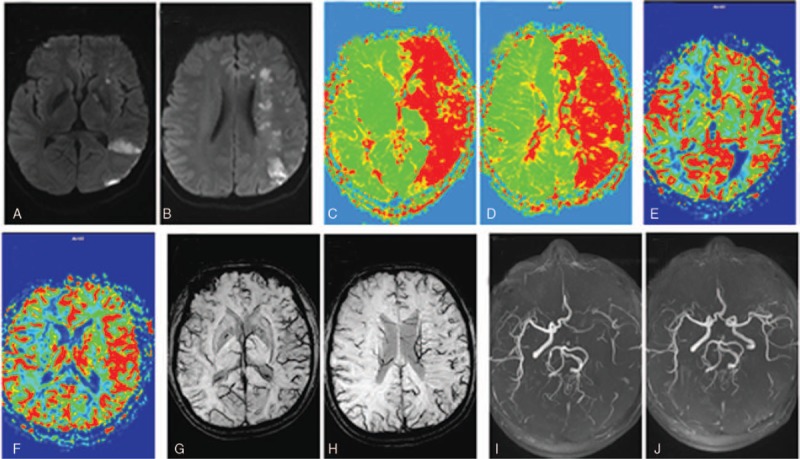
Patient experienced a cerebral infarction caused by acute occlusion of the left internal carotid artery. The admission magnetic resonance angiography (MRA) (I) and diffusion-weighted imaging (DWI) were confirmed (A and B). The patient had an National Institutes of Health Stroke scale score of 20 on admission and mean transit time (MTT) on perfusion-weighted imaging (PWI) (C and D) MTT prolonged in the area of the responsible vessel, MTT - Alberta Stroke Program Early CT score (MT-ASPECTS) was 2 points; cerebral blood volume (CBV) in the PWI showed (E and F) CBV in the lesion area increased compared with the healthy area CBV, CBV-ASPECTS was 1 point; in the susceptibility-weighted imaging (SWI) (G and H). The presence of a large number of small and prominent blood vessels (PV) in the same region, SWI-ASPECTS is 2 points; review of the head after treatment MRA (J) prompted the left internal carotid artery to achieve recanalization, mRS for 90 days after 2 points, the prognosis Better, reflected on SWI, PV grade = 2, suggesting that the cortex pia mater is better in the patient and the patient has an effective revascularization.
